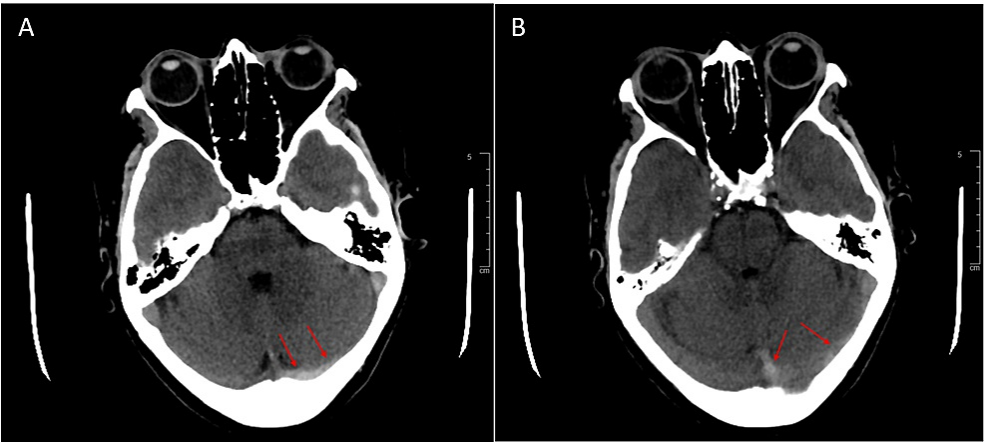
A-B: Noncontrast CT head demonstrating a hyperdensity within the left transverse sinus and left sigmoid sinus.
